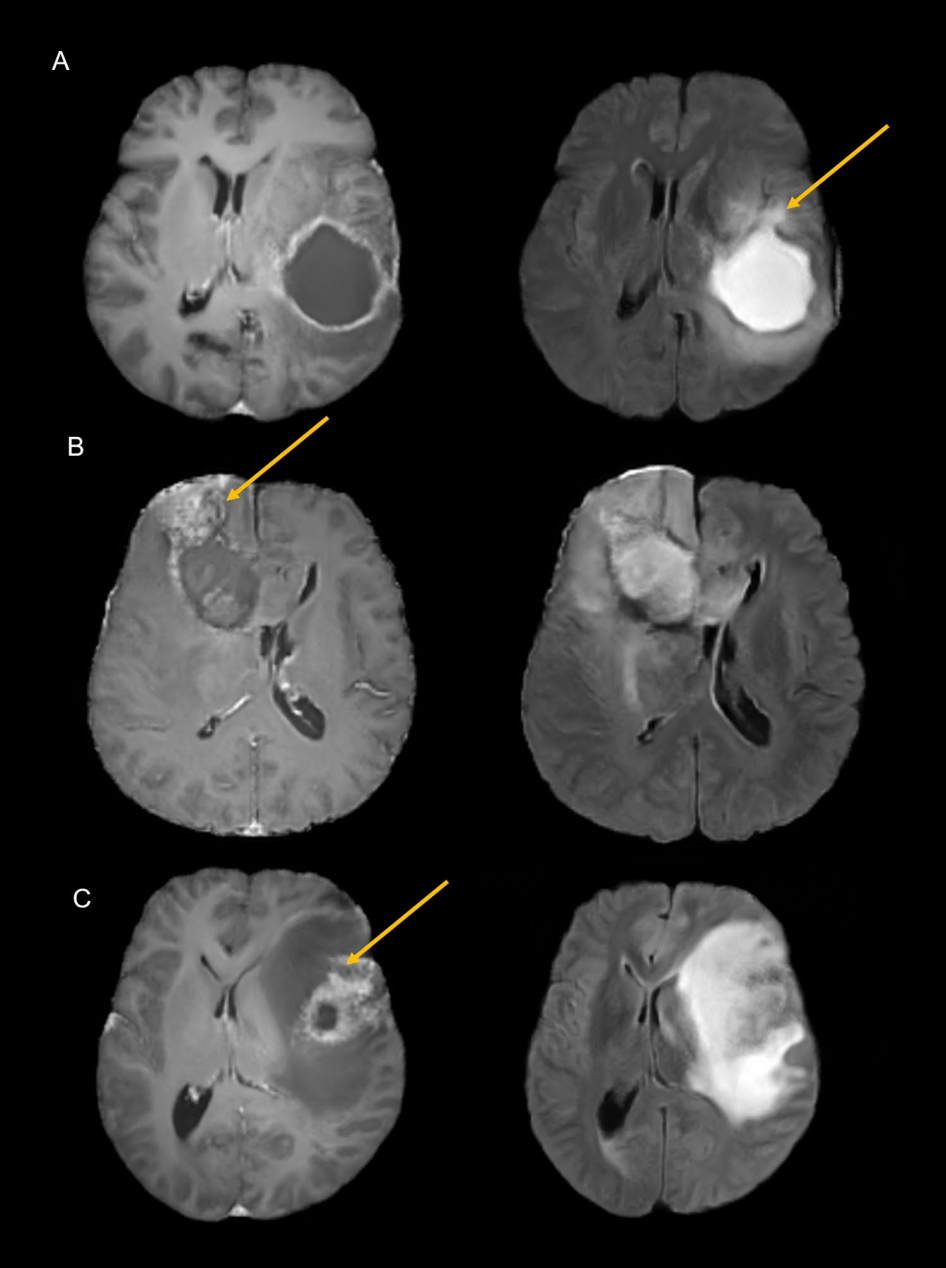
Representative synthetic images correctly determined to be synthetic by neuroradiologists. (A) Contrast-enhanced T1-weighted (CE-T1w) image similar to a real image, coupled with a FLAIR image showing an open rim of hypointensity, suggesting that the image was not real. (B) CE-T1w images showing nodular enhancement with a mesh-like artifact, suggesting that these images were not real. (C) CE-T1w images showing bizarre-shaped linear enhancement, suggesting that these images were not real.
